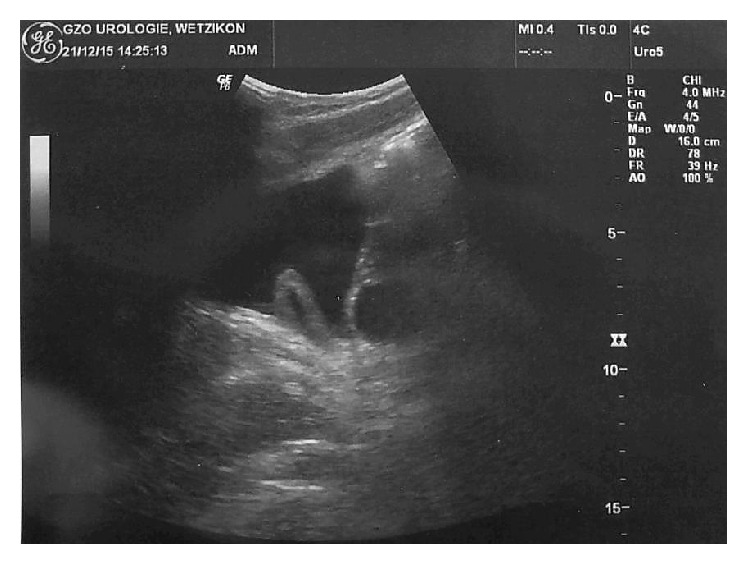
Sagittal section of the sonographic control at the 12-month follow-up. The ureterocele has significantly downsized compared to the initial CT scan (Figure 3).
